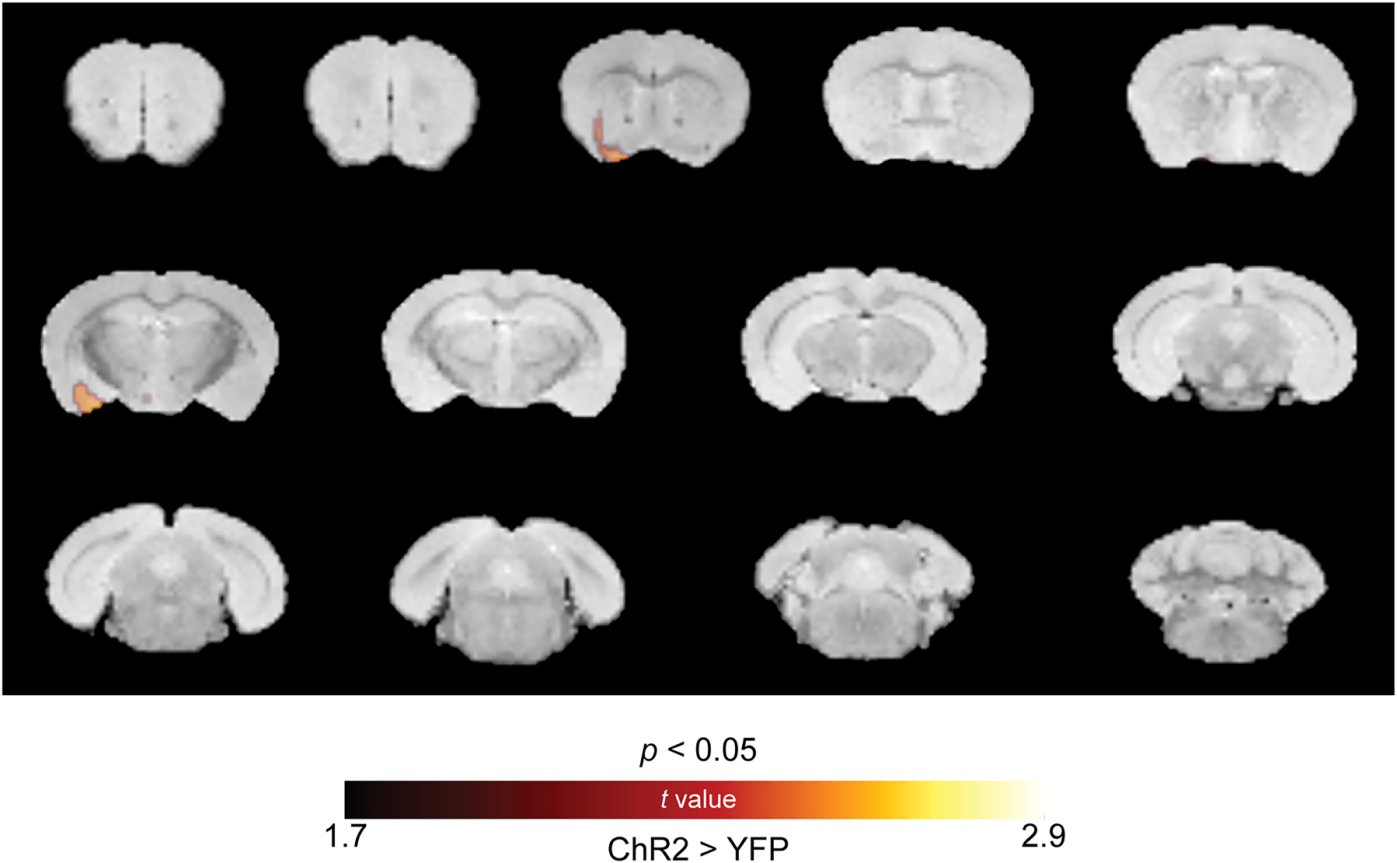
Photoactivation of the VMH→AHA pathway increases neuronal activity in the amygdala and ventral striatum. FDG-PET overlaid on structural MRI shows that VMH→AHA stimulation significantly increases FDG uptake compared with controls (*p* < 0.05, unpaired *t* test; *n* = 6 ChR2 and 10 YFP) in a region encompassing the lateral/ventrolateral striatum (left) and amygdala (right). Statistical tests are detailed in Extended Data Table 1-1.
